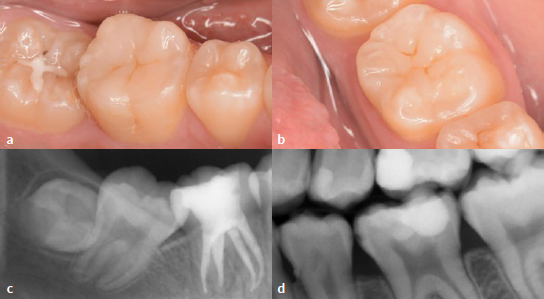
One-year follow-up. Intraoral views of teeth 46 (a) and 36 (b). (c) An apical radiograph of the restored tooth 46. (d) A bitewing showing the restoration of tooth 36.
